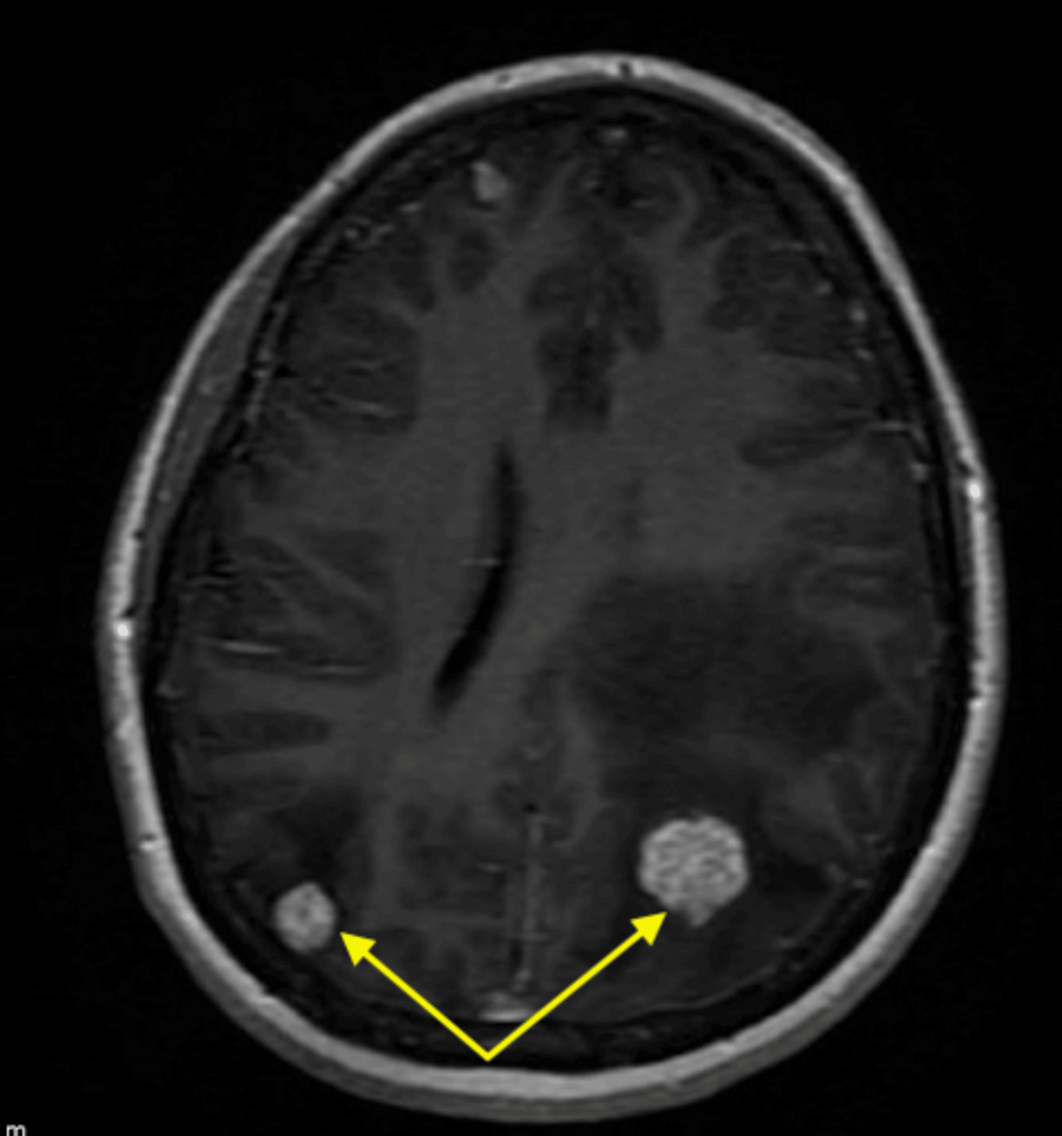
Axial T1-weighted post-contrast MRI showing enhancing lesions in the right posterior parietal and left parietal lobes (yellow arrows) with surrounding vasogenic edema.
